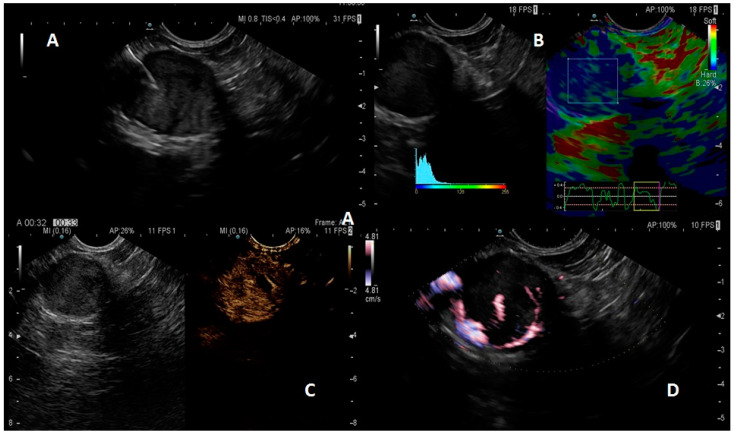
Pancreatic neuroendocrine tumor (pNET) aspect. B-mode (A): hypoechoic lesion with well-defined margins; qualitative and semi-quantitative elastography; (B): pNET as a stiff, blue lesion; pNET at intravenous contrast; (C): early hyperenhancement of the contrast; pNET aspect at power Doppler (D), with a peripheral vessel capsule.
